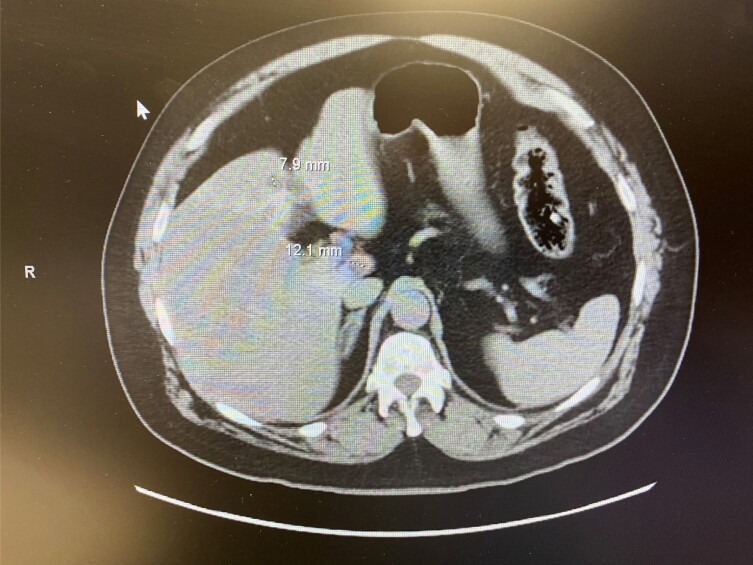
Post treatment CT, 2 years after Mefloquine and Mebendazole treatment.
